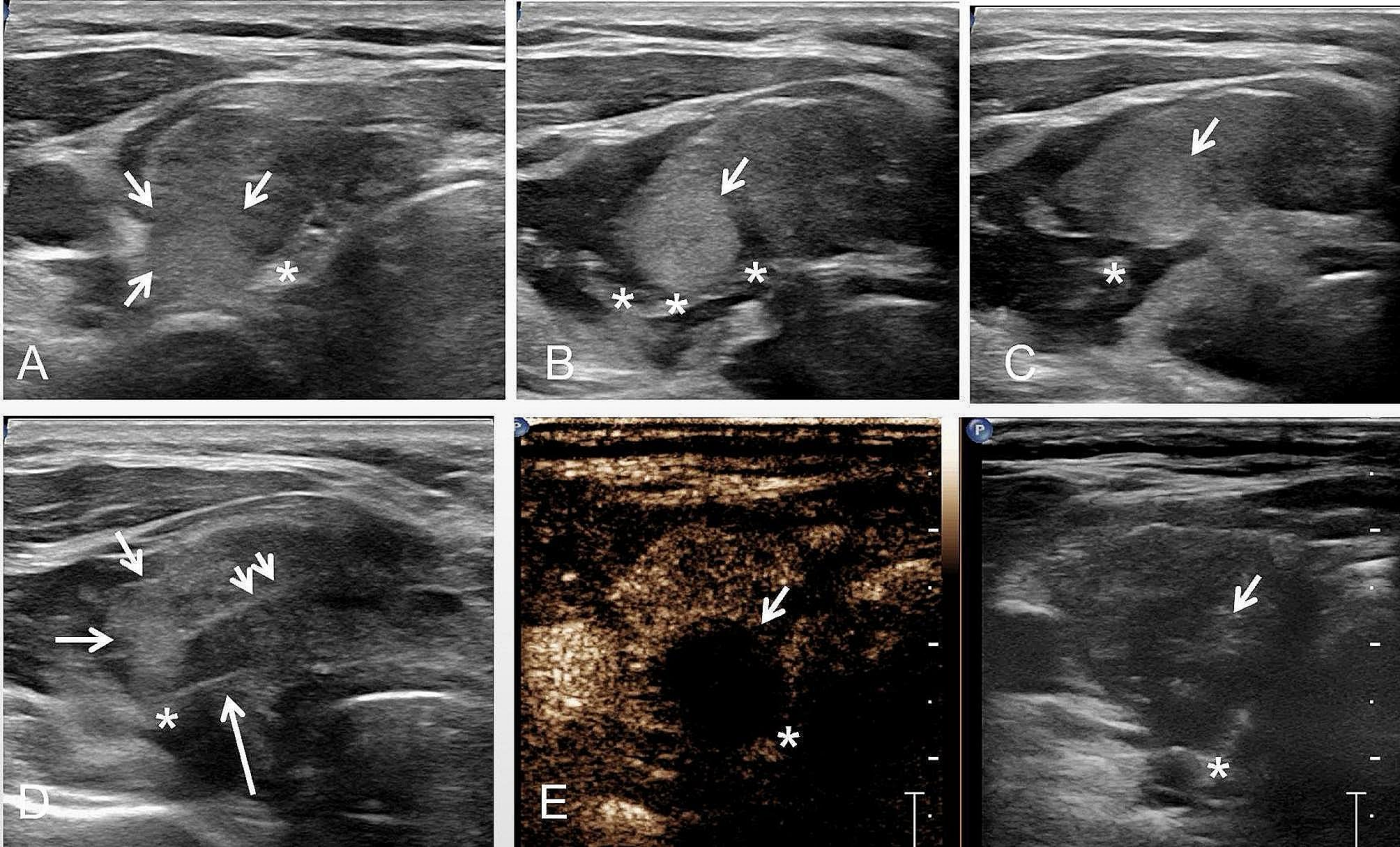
A 45-year-old female patient with nodular goiter in the left lobe of the thyroid underwent US-guided MWA. (A) The relationship between the RLN (asterisk) and the ZTTN (white arrow). The distance from the farthest outside of ZTTNs (white arrow) to the outer edge of thyroid is 4.0 mm (ZTTN grade 1). The RLN (asterisk) is located at the side of ZTTN (white arrow), and the relationship between ZTTN and RLN is Type D. (B) shows the relationship between the ZTTN (white arrow) with the RLN (asterisk) in the long axial section after the hydrodissection. (C) After the physiological saline solution was injected, the distance between the RLN and ZTTN significantly widened in the short-axis section. (D) The ablation antenna (double short white arrow) was placed inside the ZTTN (white arrow), and the injection needle (long white arrow) was placed between the ZTTN (white arrow) and RLN (asterisk). During the microwave ablation, the physiological saline solution could be continuously injected between the ZTTN (white arrow) and RLN (asterisk) to protect the RLN. (E) After MWA, the contrast-enhanced ultrasonography showed no enhancement in arterial and venous phase of ZTTN (white arrow), but high enhancement in RLN (asterisk), suggesting that RLN was not damaged during ablation
